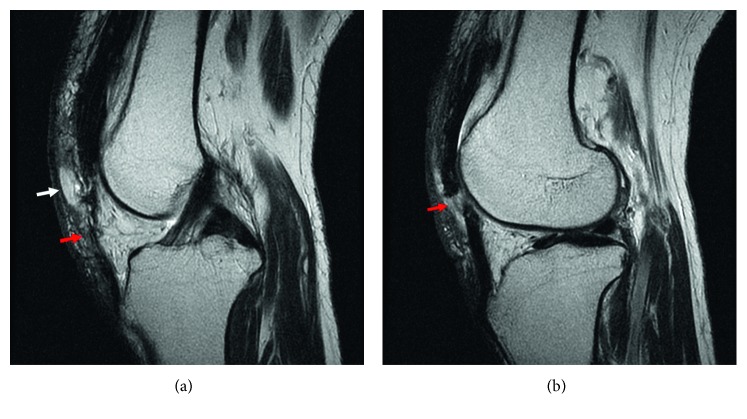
T2-weighted magnetic resonance imaging showed a loose patellar tendon (red arrow) and subcutaneous edema (white arrow) (a) and tendon disruption (red arrow) (b) at the proximal side of the patellar tendon.
